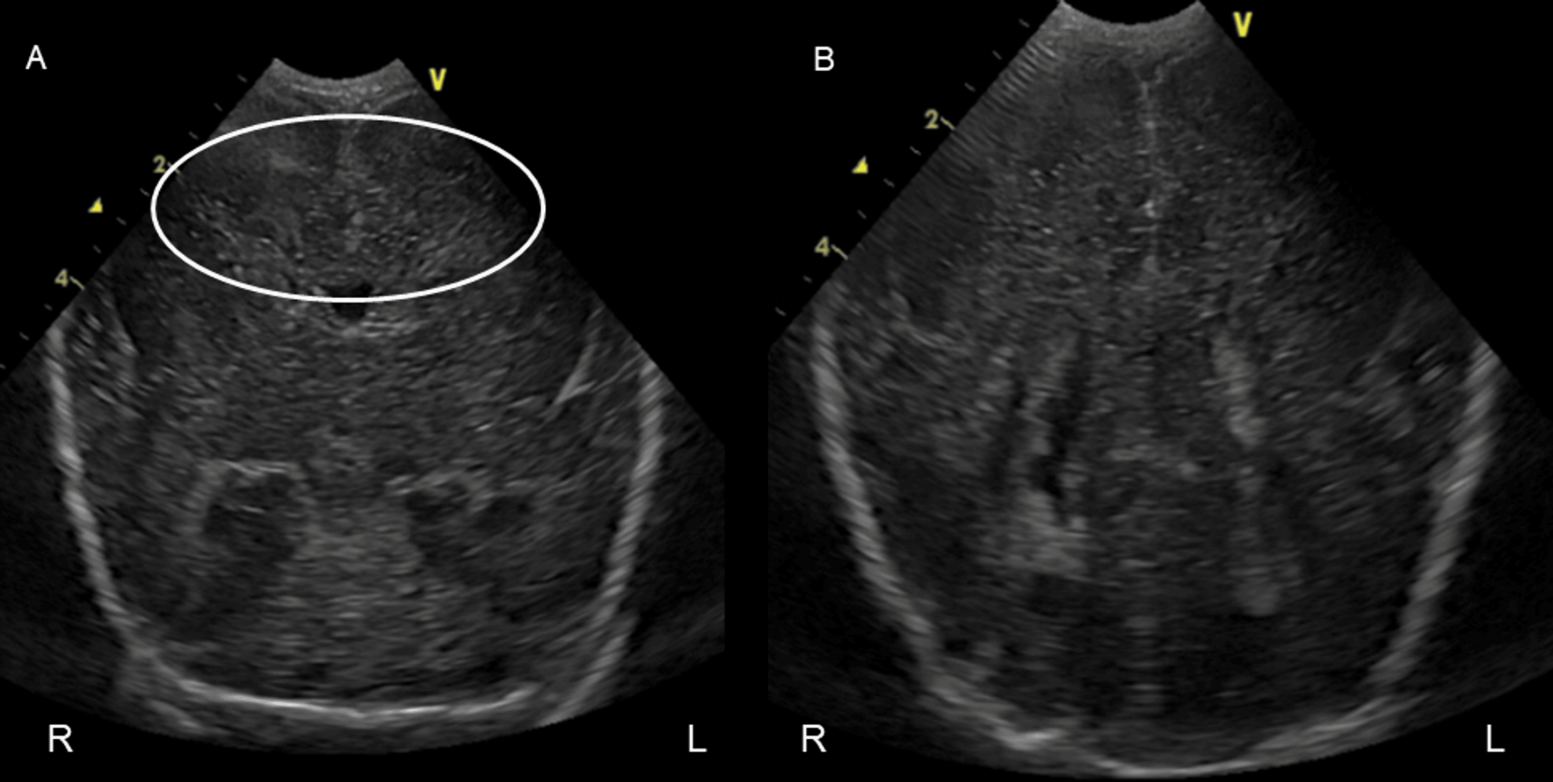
Coronal ultrasonography on the day zero of life R: right, L: left. Retrospective assessment indicated the absence of sulci corresponding to the cingulate gyrus (indicated by the white circle in A).
